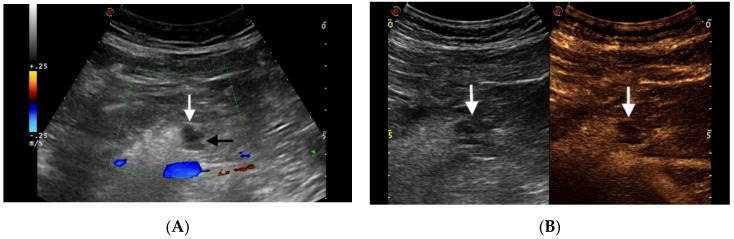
Branch duct IPMN. (A) Color Doppler Ultrasonography (dotted box) depicts lack of vascularity within a 1 cm anechoic cystic mass (white arrow) in the pancreatic body. A tiny septum is also appreciable within the lesion (black arrow). (B) On CEUS the lesion shows lack of vascularity throughout the different phases, as well as the internal septum (arrows).
